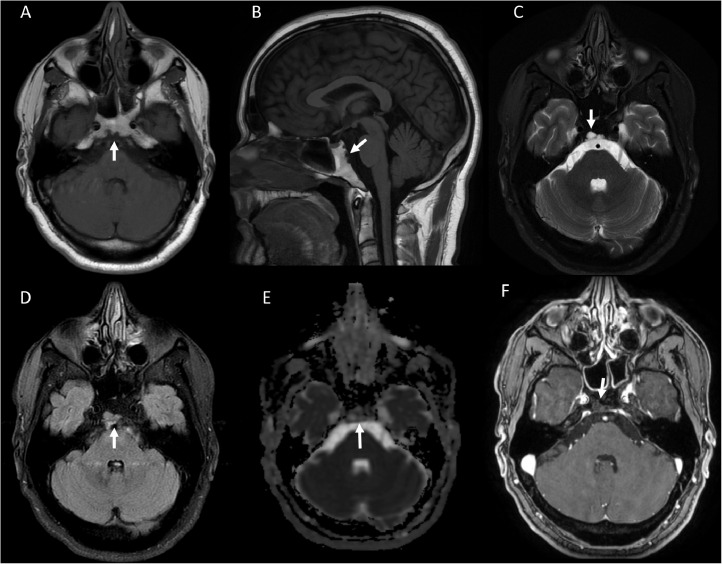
MRI brain with and without contrast was performed at our facility. This demonstrated a well-defined midline retroclival 5 mm x 12 mm cystic lesion (arrow) with hypointense signal on T1-weighted (A, B) and hyperintense signal on T2-weighted images (C), and followed CSF signal on T2-Fluid-attenuated inversion recovery (T2-FLAIR) images (D). Further, this lesion did not restrict diffusion (E) and did not demonstrate abnormal enhancement (F). Based on the midline location and characteristic imaging appearance, the findings were reported as compatible with Ecchordosis physaliphora, a benign congenital hamartomatous lesion originating from nodal cord remnants requiring no additional follow-up imaging or intervention
